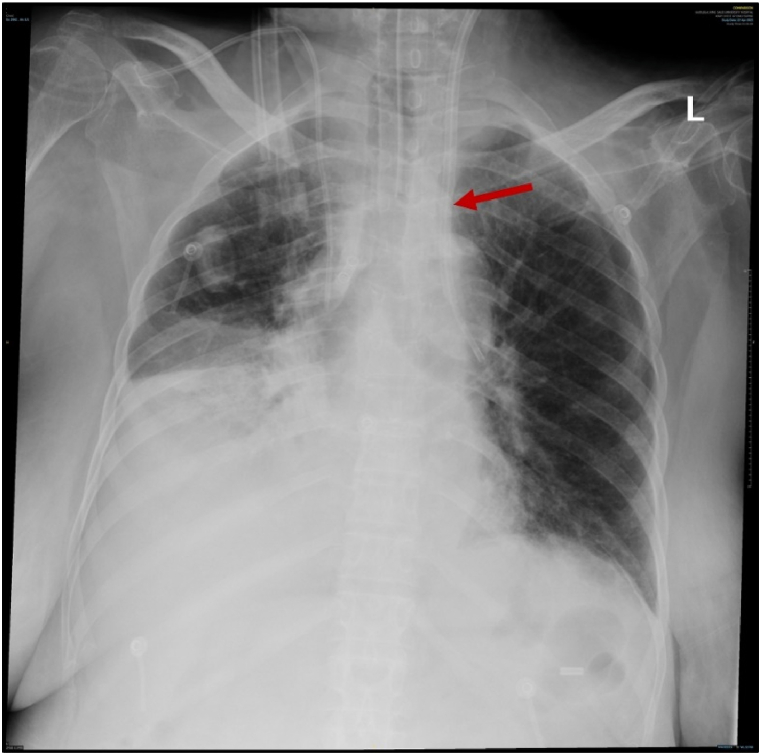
Chest X-ray demonstrating the left IJV central dialysis catheter abnormally located in the left mediastinum as indicated by the red arrow.
